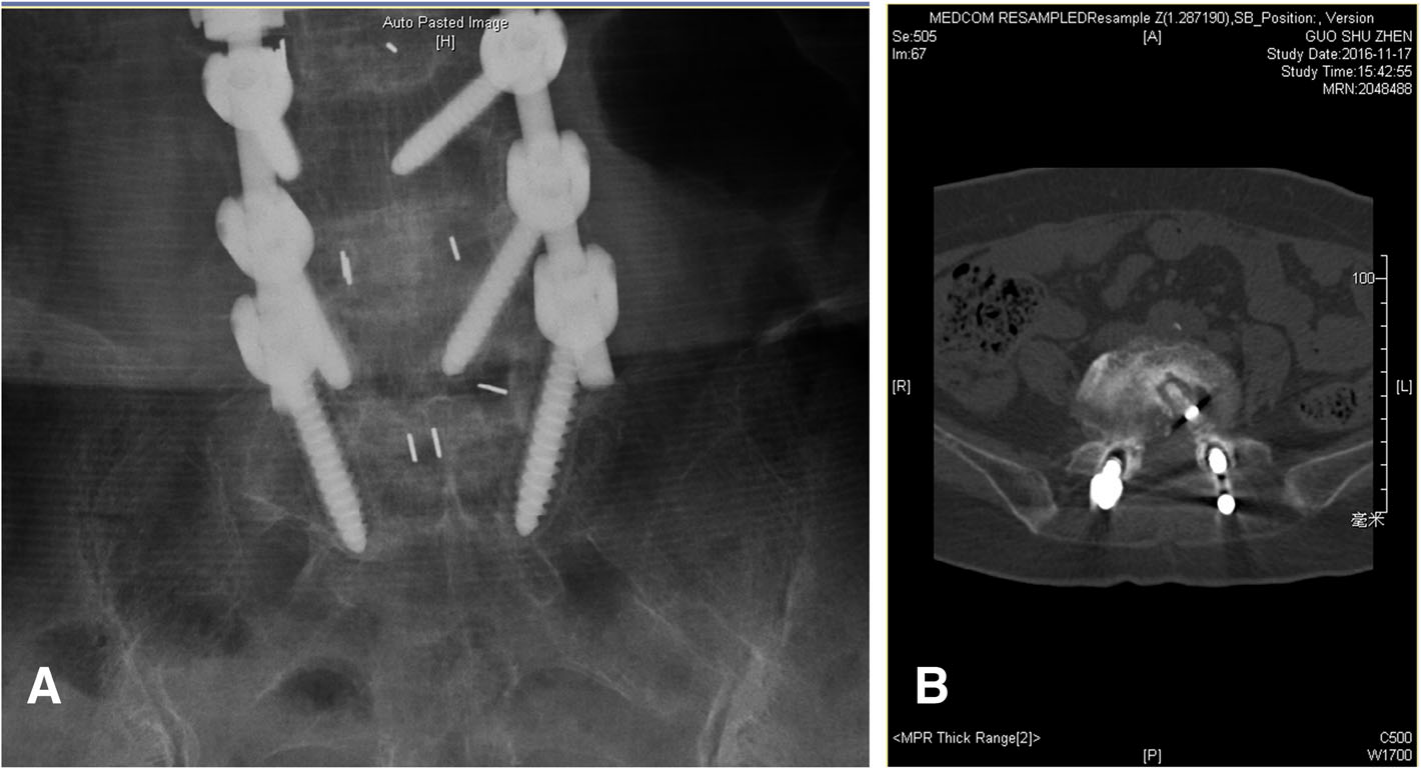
Halo sign around screws on X-ray (A) and CT (B)
